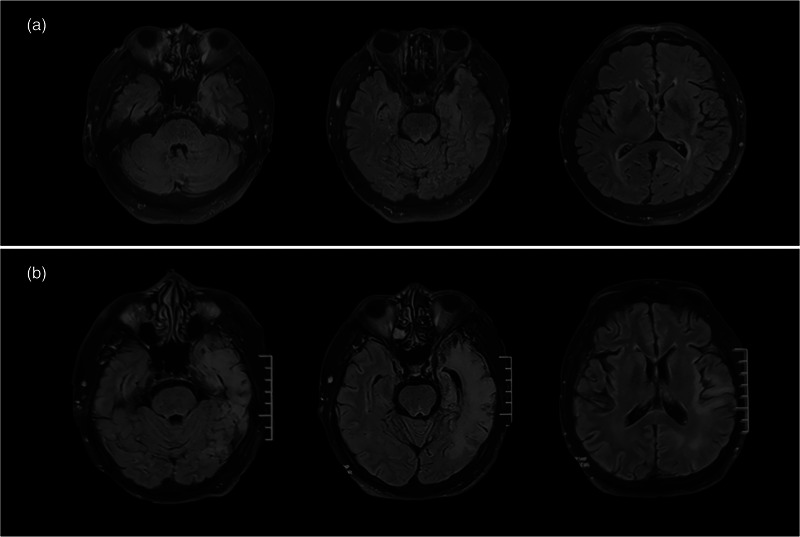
Brain Magnetic Resonance Imaging of Case 1. (a) Abnormal fluid‐attenuated inversion recovery(FLAIR) signals in the left medial temporal lobe at first admission of Case 1. (b) Expanded FLAIR hyperintensities in the left temporal, occipital, and insula lobes at second admission of Case 1.
